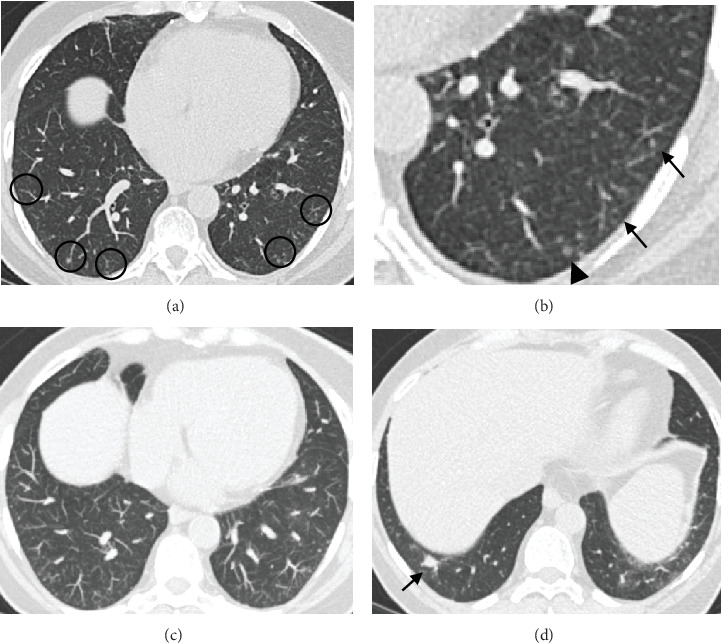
(a) Axial noncontrast chest CT (1-mm slice thickness) image shows innumerable bilateral tiny nodules of varying size, shape, and densities, predominantly in clusters in the lung bases and near the pleural surfaces (circles). (b) Axial image zoomed in the left lower lobe better demonstrates the nodules with varying size and densities (arrows), one of which has central lucency (arrowhead). (c) Axial image reconstructed with a 3-mm slice thickness using maximum intensity projection (MIP) shows distribution of the nodules in clusters near the pleural surface, sparing the pleura. (d) Axial image at the lung bases shows the dominant 1 cm solid pulmonary nodule in the right lower lobe (arrow).
